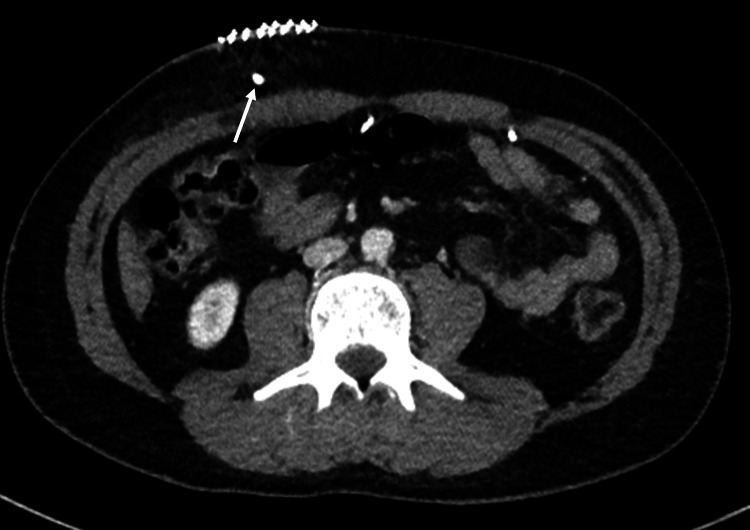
CT of the thorax, abdomen, and pelvis. White arrows: ventriculoperitoneal shunt.
